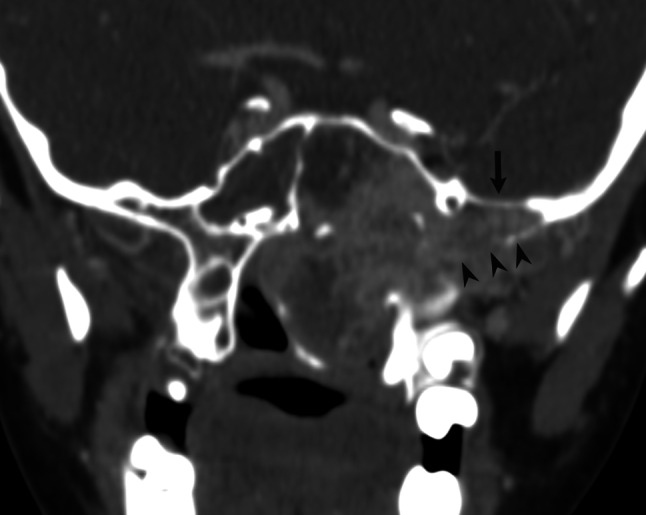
Coronal CT scan indicates extensive involvement and expansion of the pterygoid base and greater sphenoid wing diploë (*arrowheads*) with erosion of the skull base (*arrow*)
